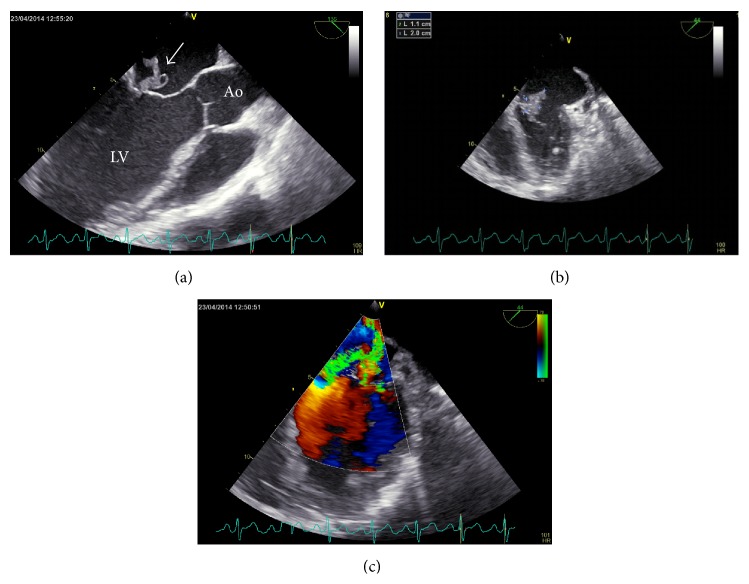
Transesophageal echocardiography (TEE): (a) 3-chamber view of the heart: floating vegetation (white arrow) on the anterior mitral leaflet; Ao = aorta; LV = left ventricle. (b) Another projection of the floating vegetation (11 mm × 20 mm) and the destroyed mitral valve. (c) Color Doppler sonography shows severe mitral regurgitation.
